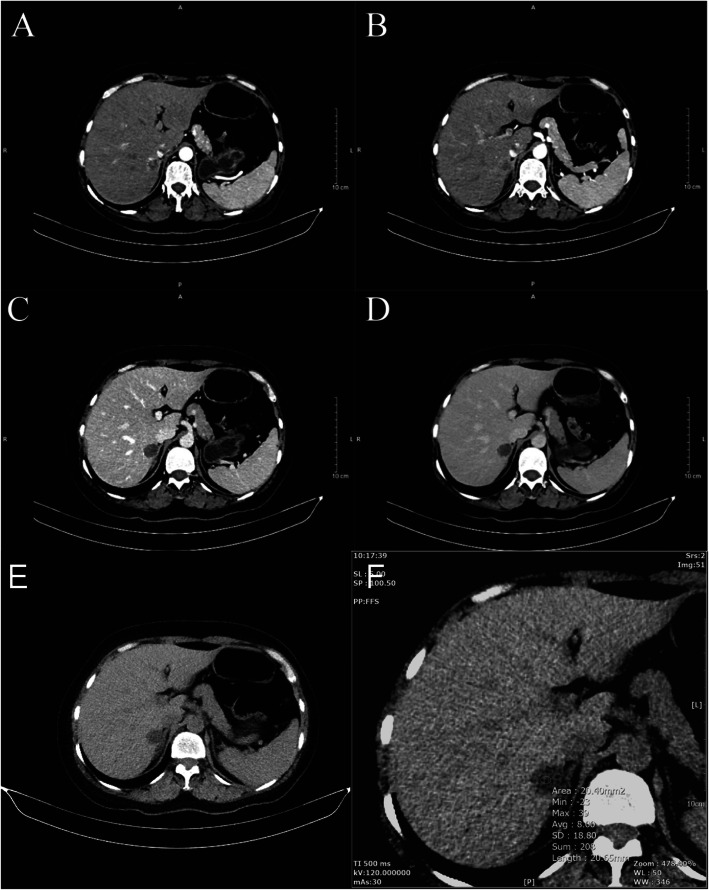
Enhanced CT of the abdomen showing an irregularly enhanced node at the seventh section of the arterial phase. The node contained edges enhanced in a circular manner and a few dense fat particles. The enhancement was evident during the venous portal and delayed stages. The focus size was 2.2 cm × 1.8 cm, showing clear boundaries. The average ordinary CT value of the tumor was 8 Hu
